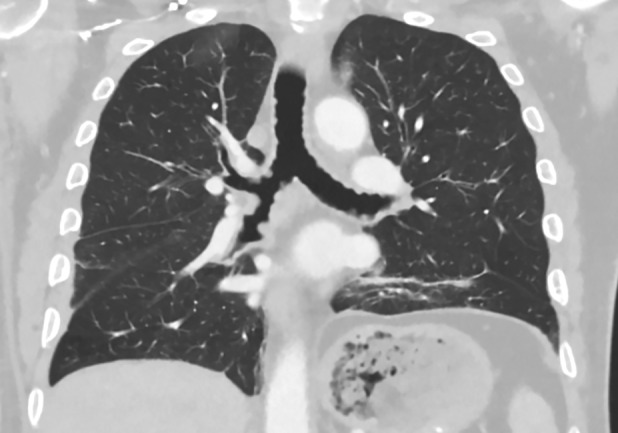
Coronal section of computed tomography (CT) of the chest (lung windows) showing multiple protruding nodularities in the tracheal bronchial lumen.
